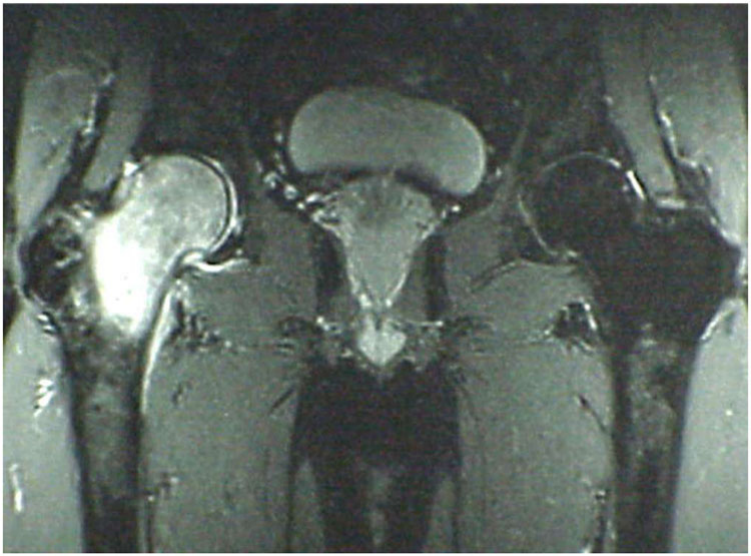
Coronal view with T2‐weighted fat‐suppressed MRI shows a high and homogenous hyperintense signal of head and femoral neck, saving trochanter, in the absence of focal abnormalities. The cortical profile is maintained, particularly the upper polar region remains spherical. No signal alterations on the acetabular side. A small intra‐articular effusion is present (1.5 T scanner; Magnetom Espree, Siemens). MRI, magnetic resonance imaging.
